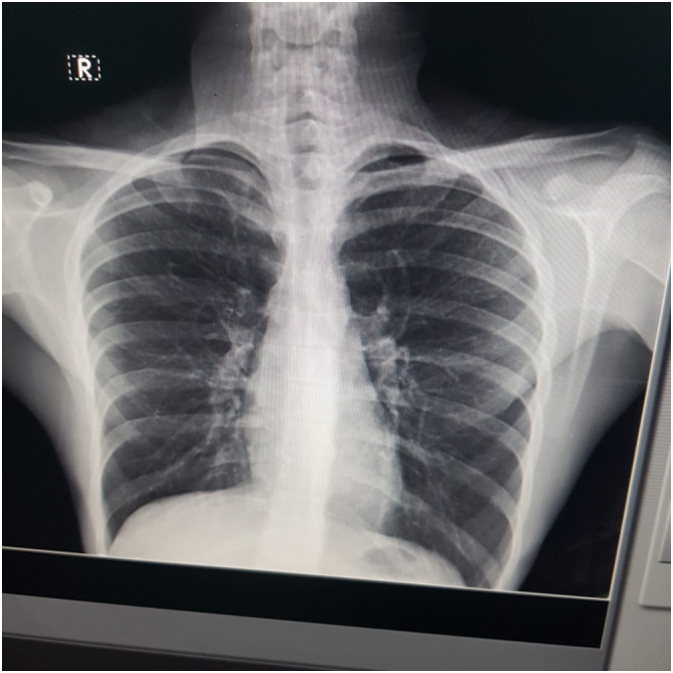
Chest X-ray performed one year ago, showed no obvious abnormalities.
